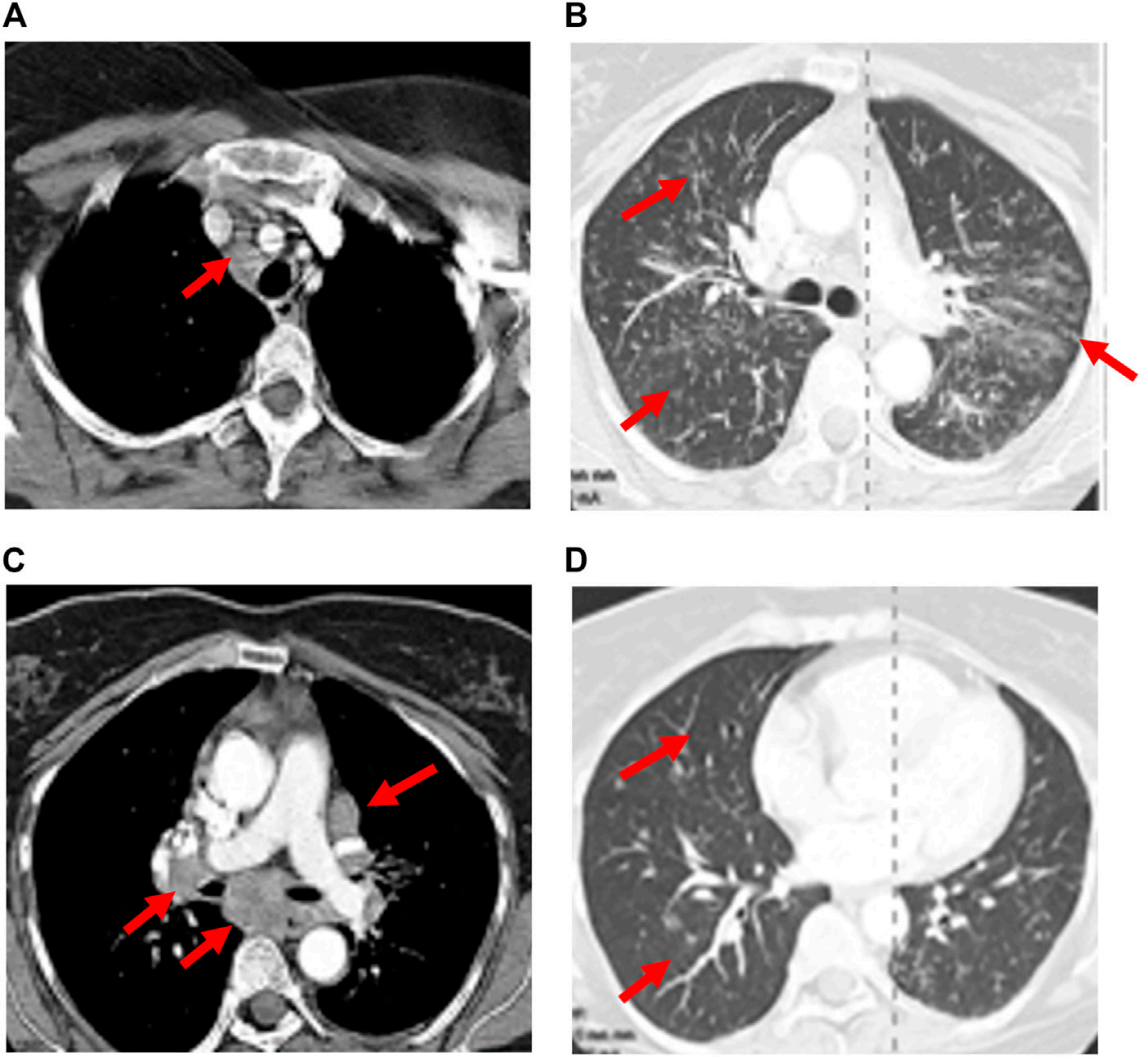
Recrudescence of pneumonitis with drug rechallenge. A 47-year-old man presented with dry cough and dyspnea 11 weeks after initiation of Nivolumab for treatment of non-Hodgkin lymphoma. CT scan showed patchy bilateral ground glass changes (A,D). Bronchoscopy with biopsy confirmed organizing pneumonia associated with grade II pneumonitis and the patient was started on high dose steroids. Clinical and radiographic improvement was seen 6 weeks later after tapering off steroids (B,E). Nivolumab was resumed. Three weeks later, the patient returned with increased respiratory symptoms and hypoxia. CT imaging demonstrated bilateral infiltrates which were worse than initial presentation (C,F).
